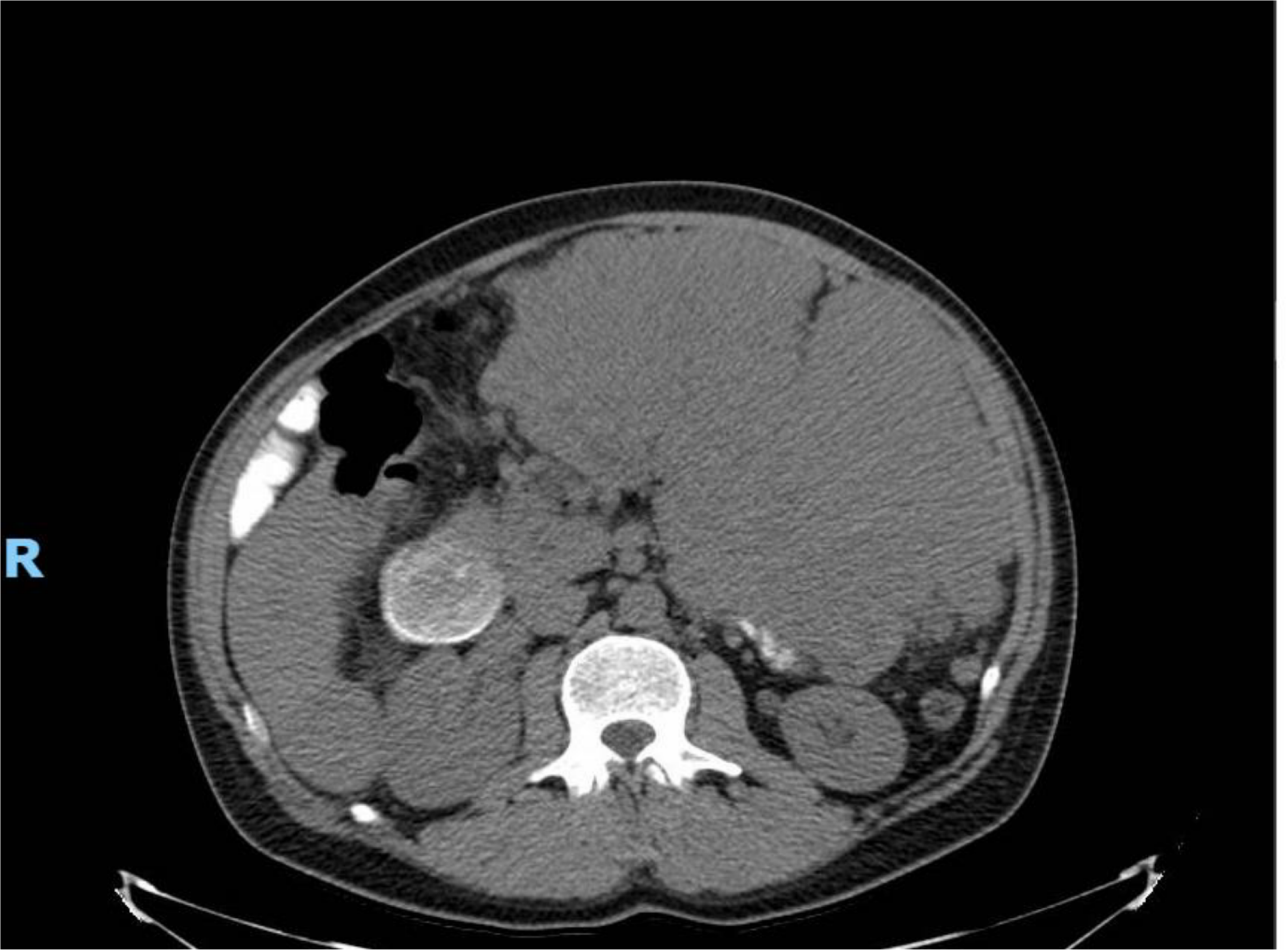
Initial CT of abdomen/pelvis with large mass, with largest component measuring 23 × 15 × 28 cm in left abdomen, with evidence of metastatic disease in the liver. Imaging notable for duodenal dilation and partial obstruction of small bowel.
